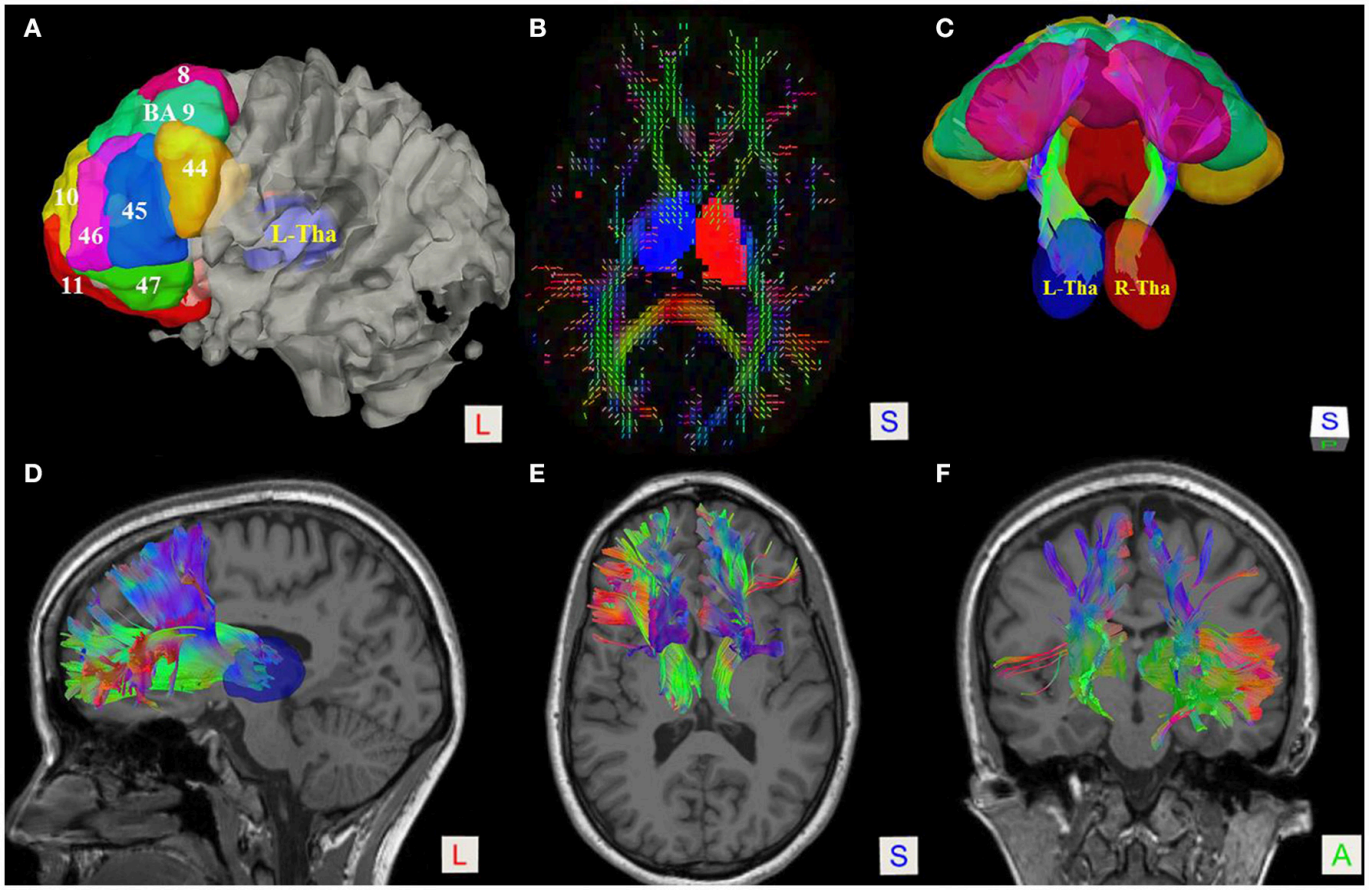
Fiber tractography of the thalamic-prefrontal peduncle (TPP; subject No. 8). (A) Seeding regions of interest (ROIs) were placed on the prefrontal cortex, including Brodmann areas (BAs) 8–11 and 44–47. The thalamus (Tha) was set as the ending ROI. The ROIs were overlaid on the white-matter surface. (B) Axial slice at the thalamus level on a quantitative anisotropy (QA) color map; Ending ROIs were placed at left thalamus (blue) and right thalamus (red). (C) Posterior-superior view of the TPP and ROIs. (D–F) Lateral, superior, and anterior views of the TPP, overlaid on T1 MRI slices.
